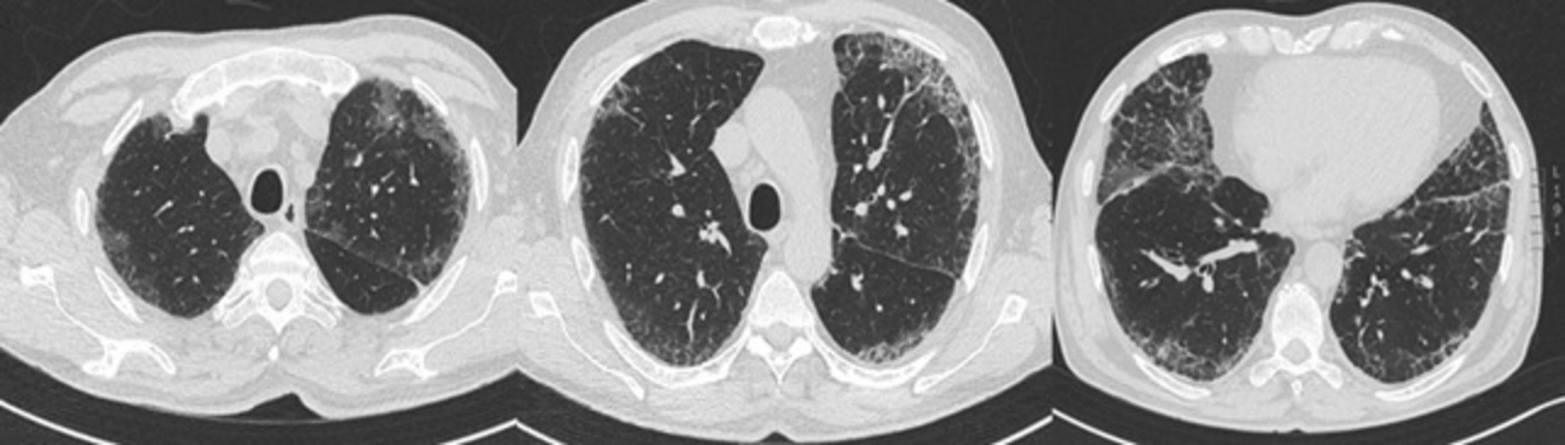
Chest HRCT scan performed at admission. The CT scan revealed bilateral fibrotic interstitial abnormalities associated with ground‐glass opacities and diffuse enlargement of mediastinal lymph nodes.
